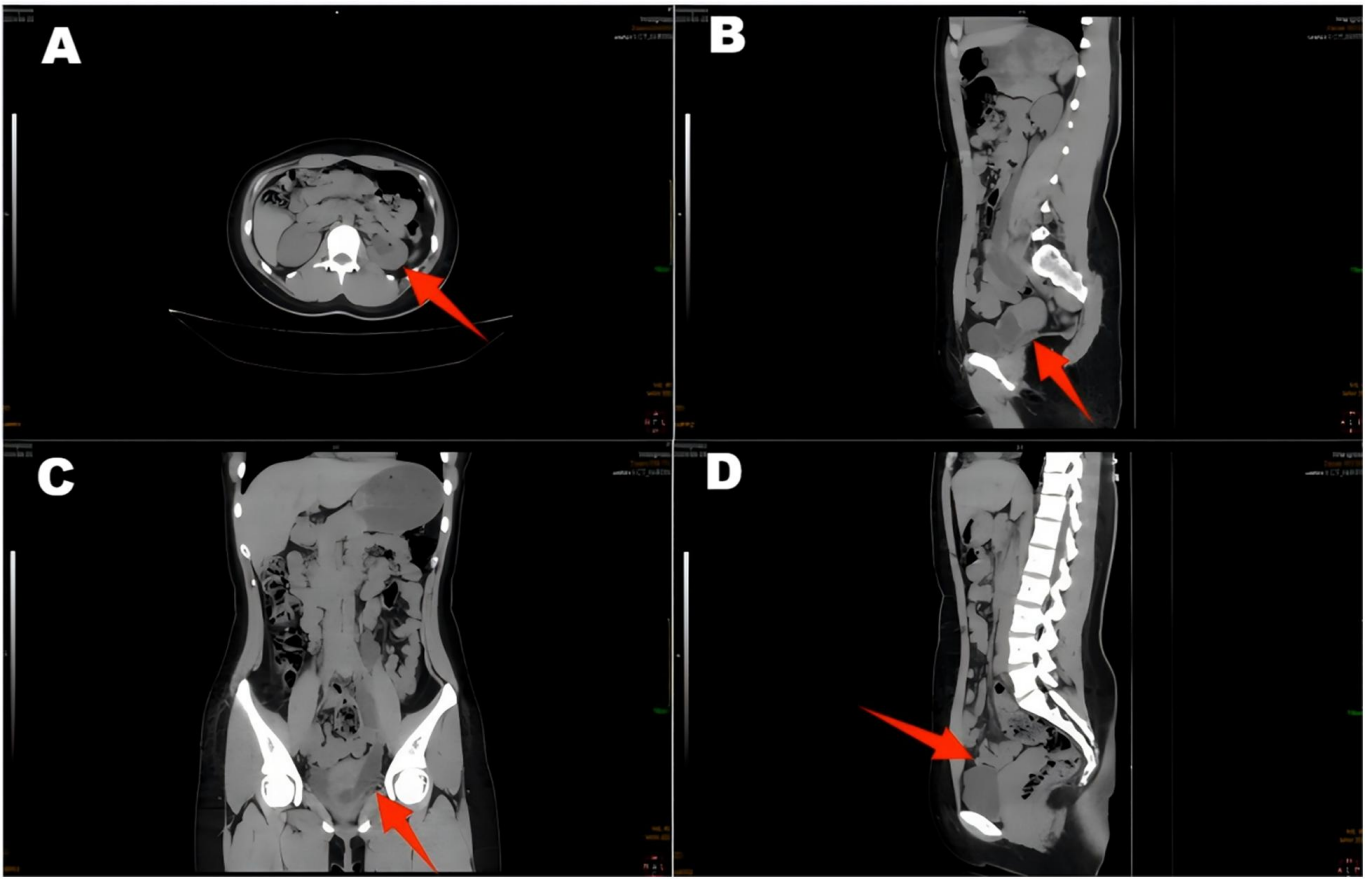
CT scan of the ureter shows hydronephrosis of the left renal pelvic ureter (A,C,D), with a suspected terminal ureter connected to a cystic hypodense shadow of the vagina (B).
